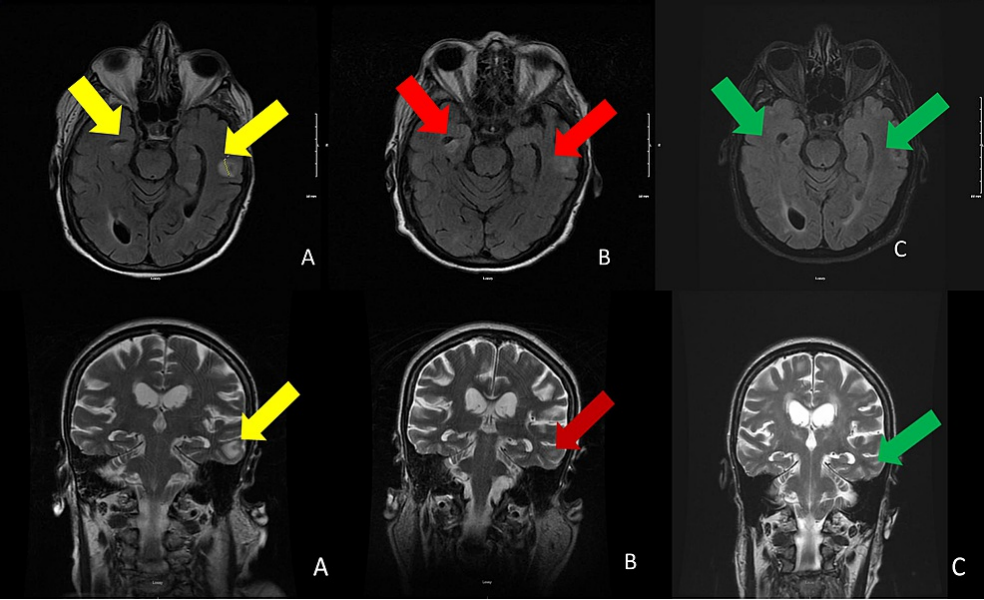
MRI of the brain without contrast, axial and coronal views A. MRI of the brain without contrast, on day five of admission, showed in the left temporal lobe with T2/fluid-attenuated inversion recovery (FLAIR) signal intensity 14 mm focus at the gray-white junction and right inferior temporal gyrus (yellow arrows). B. MRI without contrast on day 30 shows the subtle increased intensity of the right inferior temporal gyrus and decrease intensity in the left temporal (red arrows). C. MRI without contrast on day 176 shows improvement of the previous temporal and frontal enhancement (green arrows).
